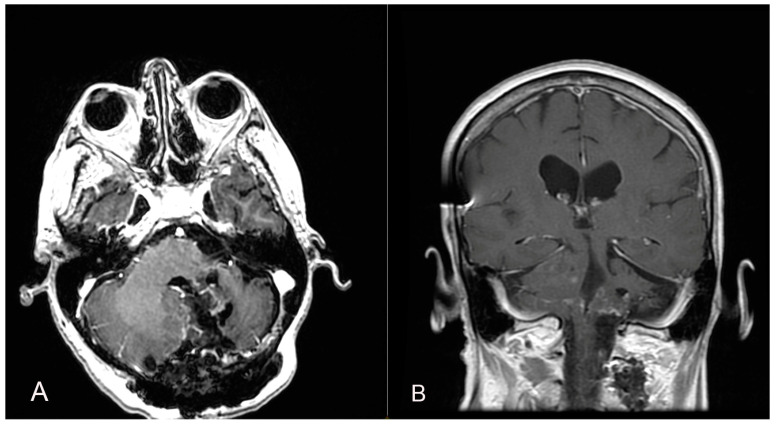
A second surgery was performed through a telo-velar approach, and postoperative contrast-enhanced brain MRI (A,B) is shown. The lesion intraoperatively appeared to be a calcified mass tenaciously attached to contiguous structures, and a portion of the tumor was found to be tightly adherent to the left lateral recess, which was left in situ after a positive irritative response during the neurostimulation of the XII cranial nerve.
